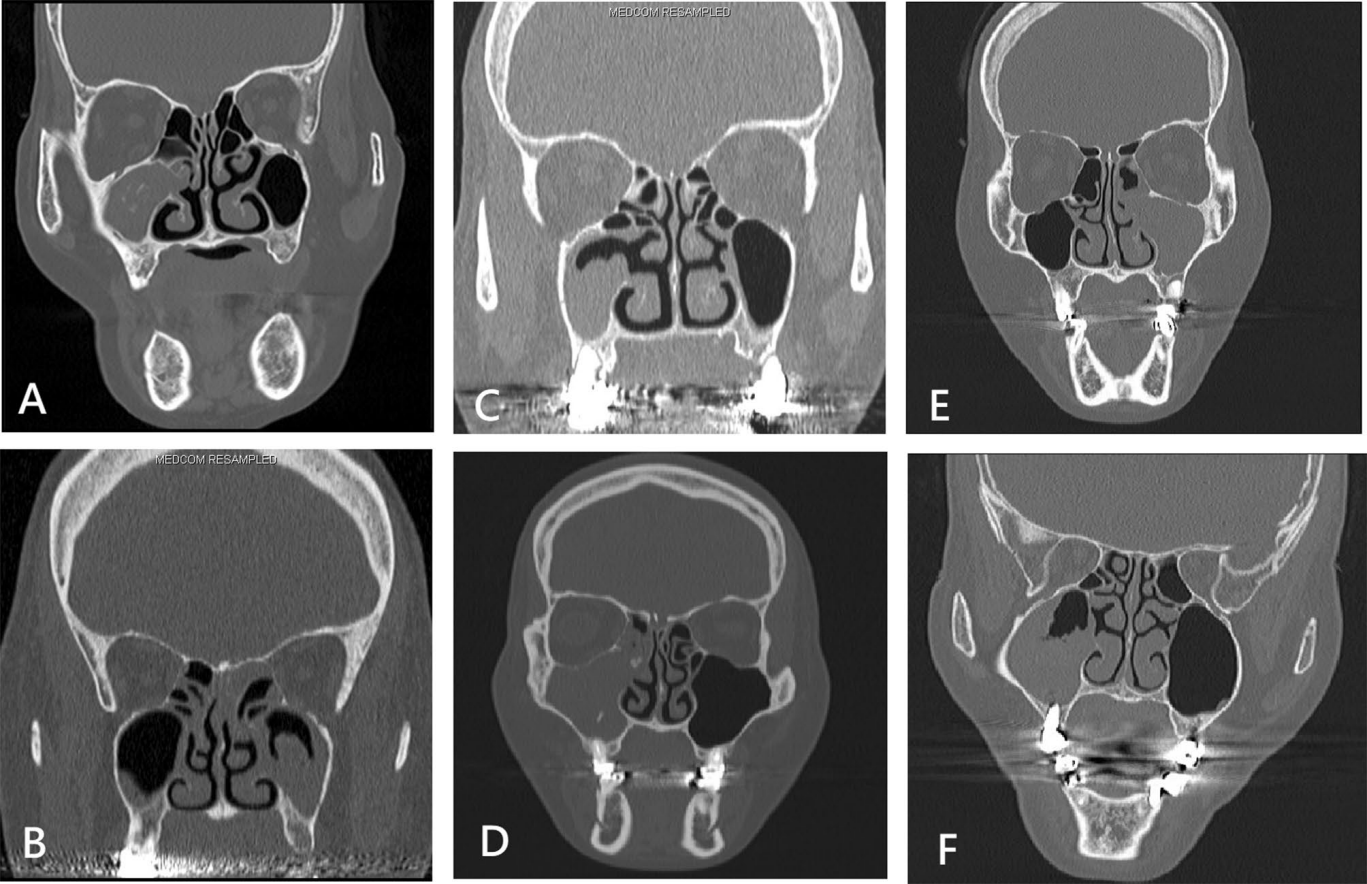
Computed tomographic features of maxillary sinus fungus ball with intralesional hyperdensity (A), without intralesional hyperdensity (B), with periodontal bone loss (C), with exogenous dental filling material (D), with periapical lucency (E), and with penetrating dental implant (F).
